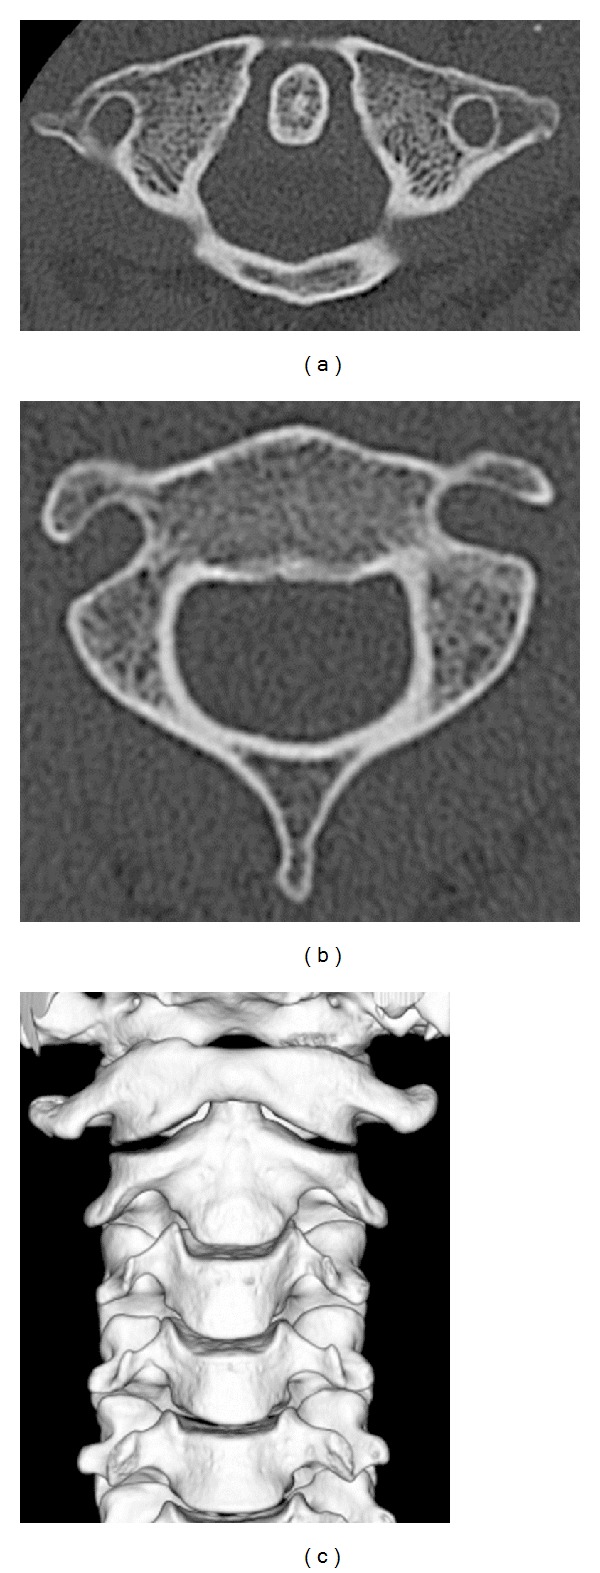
CT after reduction of atlantoaxial rotatory fixation. (a) Axial view of atlas, (b) axial view of axis, and (c) anterior view of three-dimensional CT.
